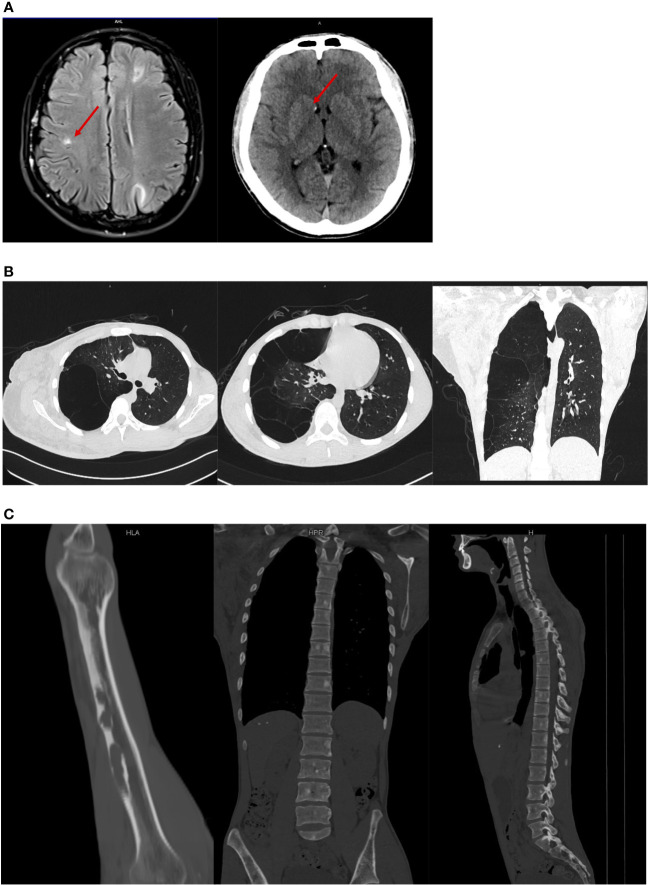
Medical imaging results. (A) Nodules showed on the head CT; (B) lymphangioleiomyomatosis on the chest CT and bone cysts in the different regions; (C) lymphangioleiomyomatosis on the chest CT and bone cysts in the different regions.
